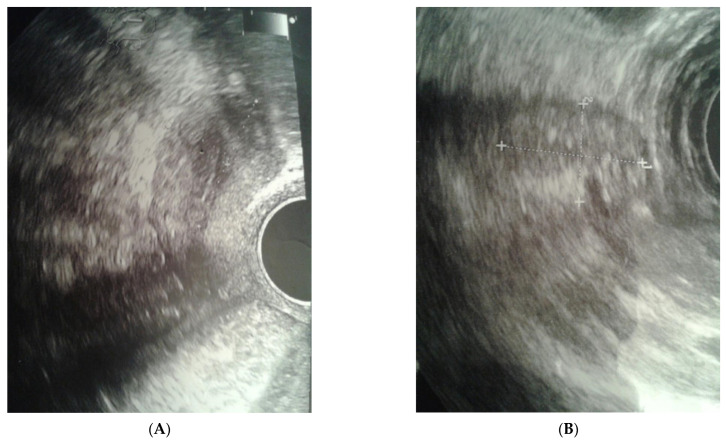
Ultrasound before surgery: (A) uterus, (B) gonad left.
